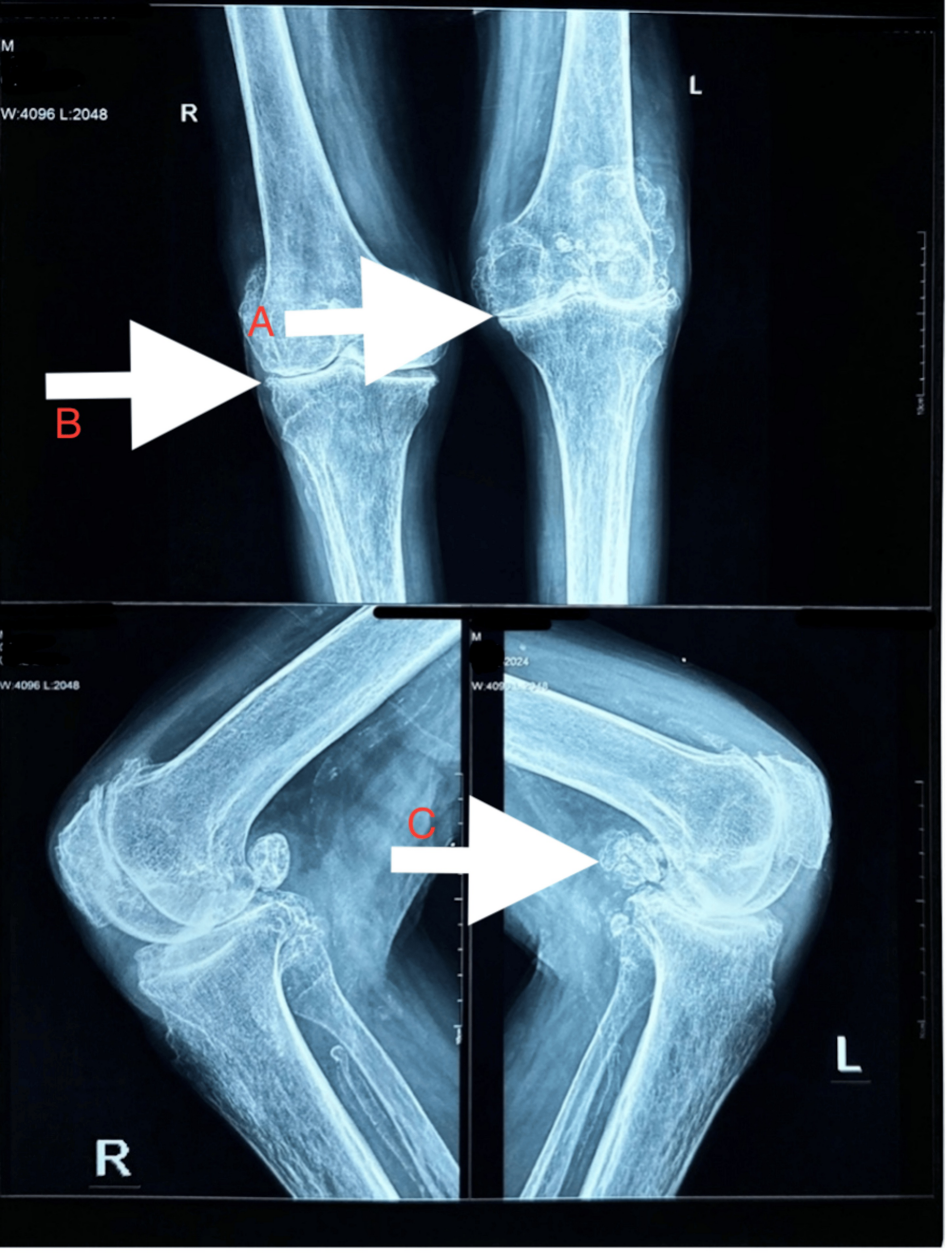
X-ray of bilateral knee (AP view above and lateral view below) showing A) joint space reduction, B) osteophyte and C) loose bodies
